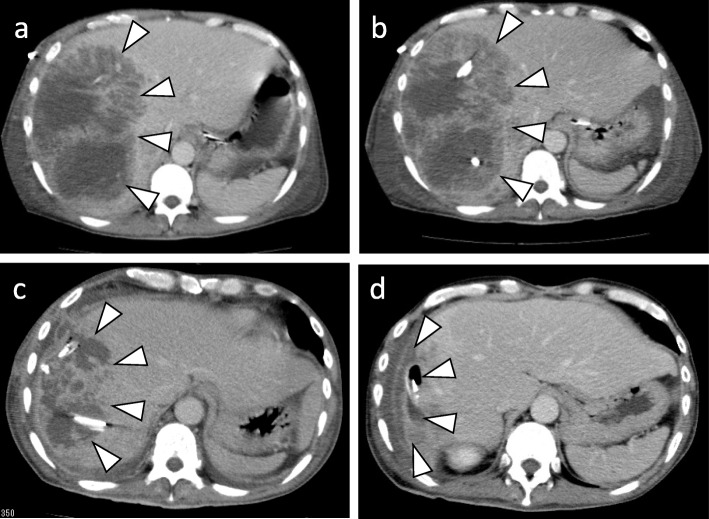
CT showing that the abscess reduced in size. a CT when the patient was admitted. b Preoperative CT. c Postoperative CT. d CT before the patient was discharged. CT: Computed tomography
